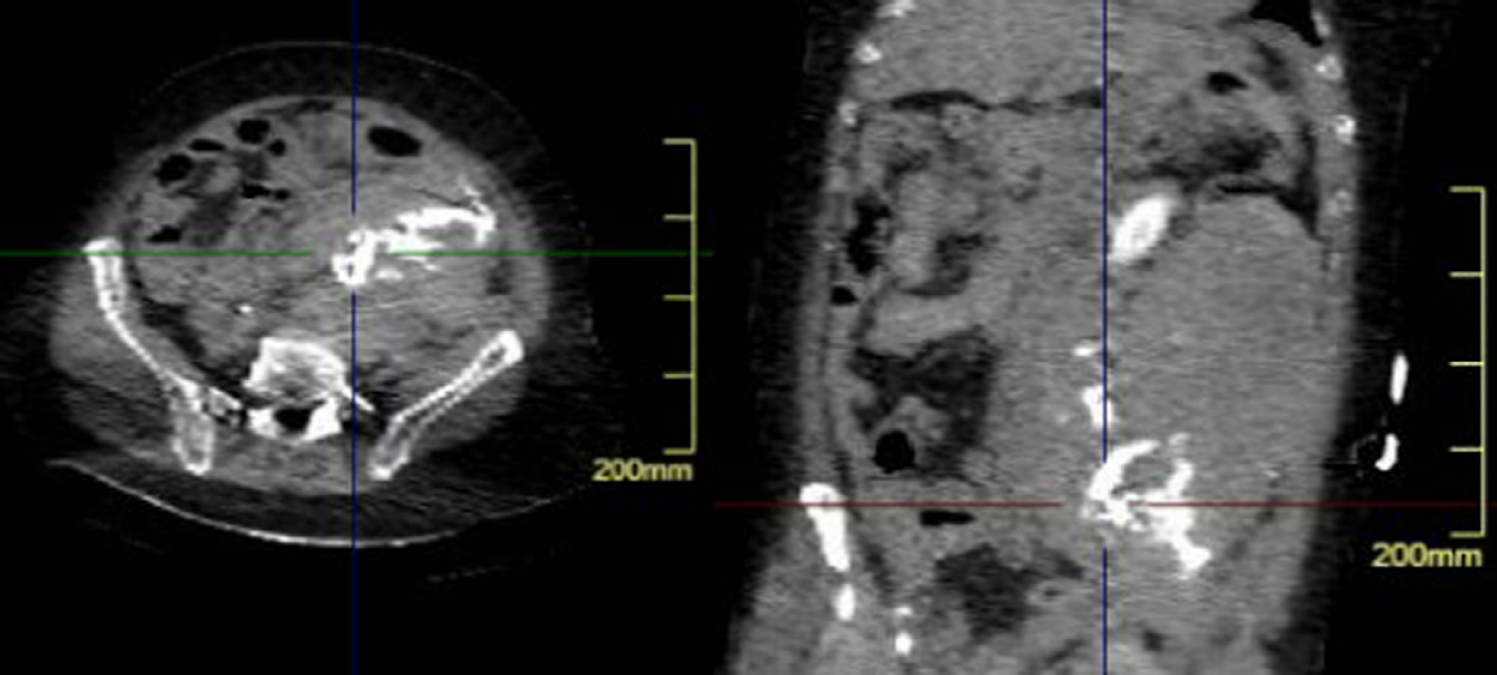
Axial and coronal view of CT scan with contrast bolus through left common femoral vein shows iliac vein rupture with contrast leakage retroperitoneal space. Notice the wide external iliac vein.
